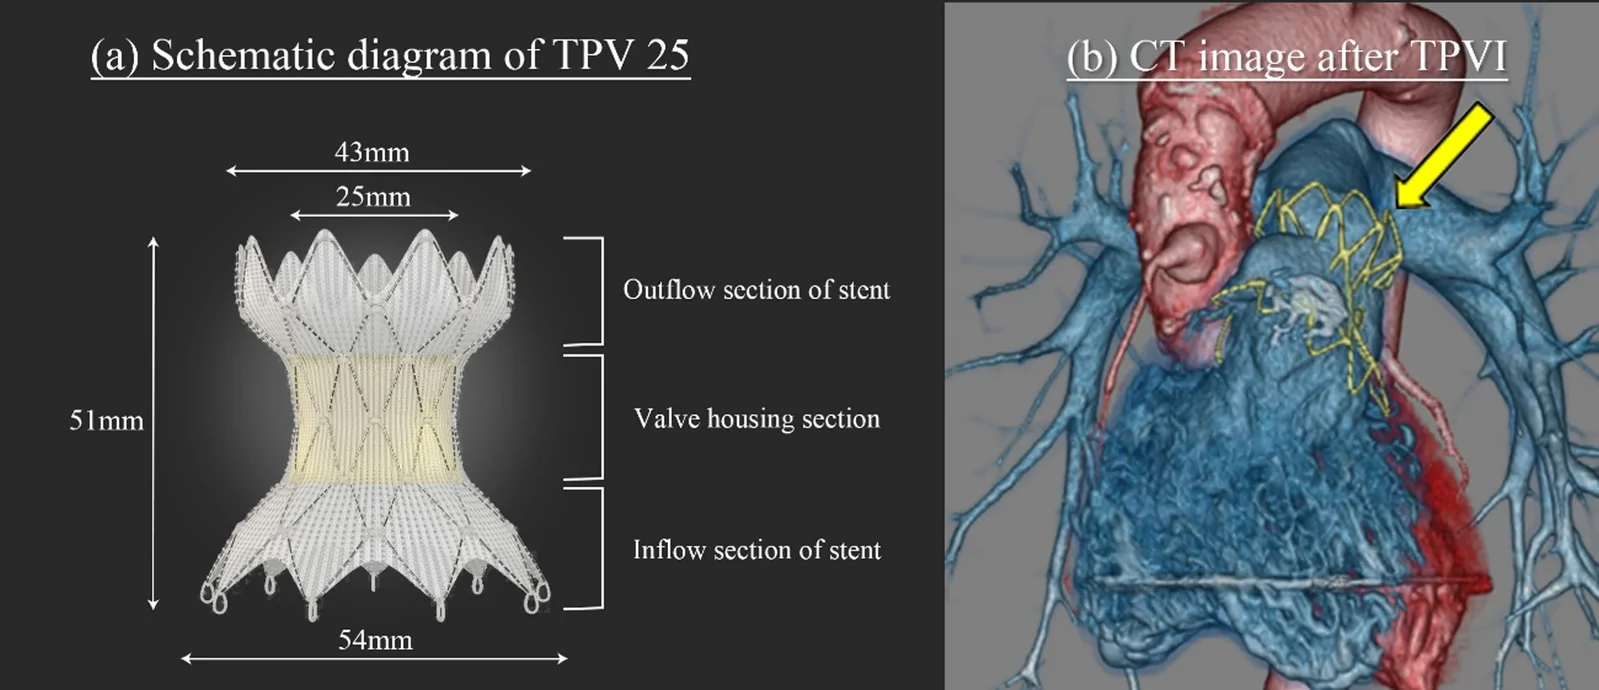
Medtronic’s Harmony TPV 25 (reproduced with permission from MEDTRONIC JAPAN CO., LTD.). (a) The bioprosthetic valve is attached in the valve housing section. (b) Post-TPVI contrast-enhanced CT image showing the Harmony 25 (arrow) positioned in the pulmonary artery. The outflow section of the stent is positioned distally in the pulmonary artery. The inflow section of the stent is placed in the right ventricular outflow tract
